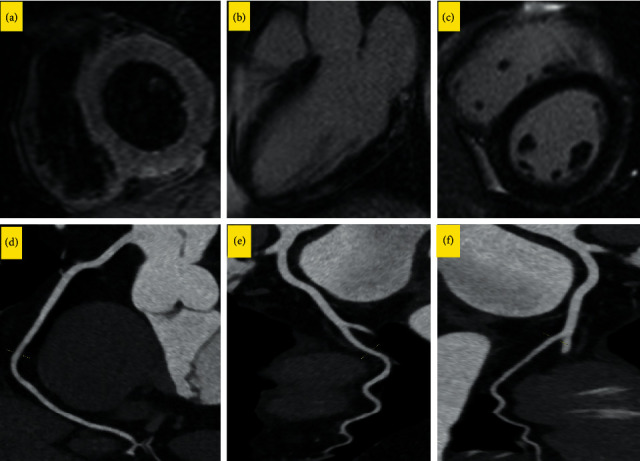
a–c) Cardiac magnetic resonance images. (a) Short-axis view short-tau inversion recovery sequence at the midventricular level demonstrates myocardial edema. (b, c) Three-chamber and short-axis LGE images depict midmyocardial-subepicardial fibrosis. (d–f) Curved multiplanar CT angiograms suggestive of normal epicardial coronary arteries.
